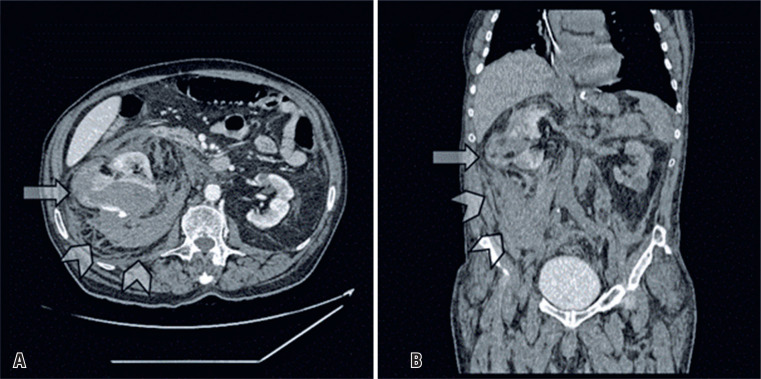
Axial (A) and the coronal (B) contrast-enhanced computed tomography images (soft tissues window) at admission showing an exophytic renal mass with a heterogeneous aspect, permeative septa, and fat-containing areas (white arrow) on the mid-pole of the right kidney, associated with perinephric and pararenal hematomas, and signs of inflammation of the adjacent fat (white arrowheads)
